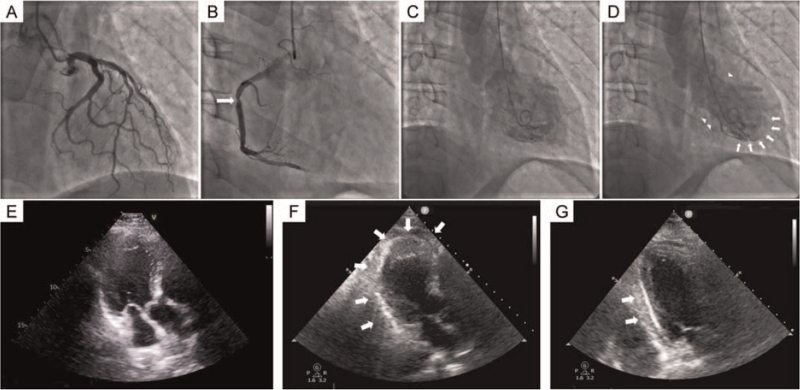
Representative changes of Takotsubo syndrome in the patient. Coronary angiography shows minor atherosclerosis in the left anterior descending artery and circumflex branch of the left coronary artery (A). Slow flow was observed in the right coronary artery, and 50% stenosis (arrow) was observed in the middle right coronary artery (B). Left ventriculography shows normal wall motion during diastole (C), but apical akinesia (arrow) with basal hyperkinesis (triangle) during systole (D). Normal echocardiography at admission (E). Bedside echocardiography shows akinesia of the apex and the left ventricular posterior wall (arrow) at the onset of Takotsubo syndrome (F). On day 4 after onset, only slight akinesia in the mid-segment of the left ventricular posterior wall (arrow) with a normal ejection fraction of 70% (G) can be seen.
